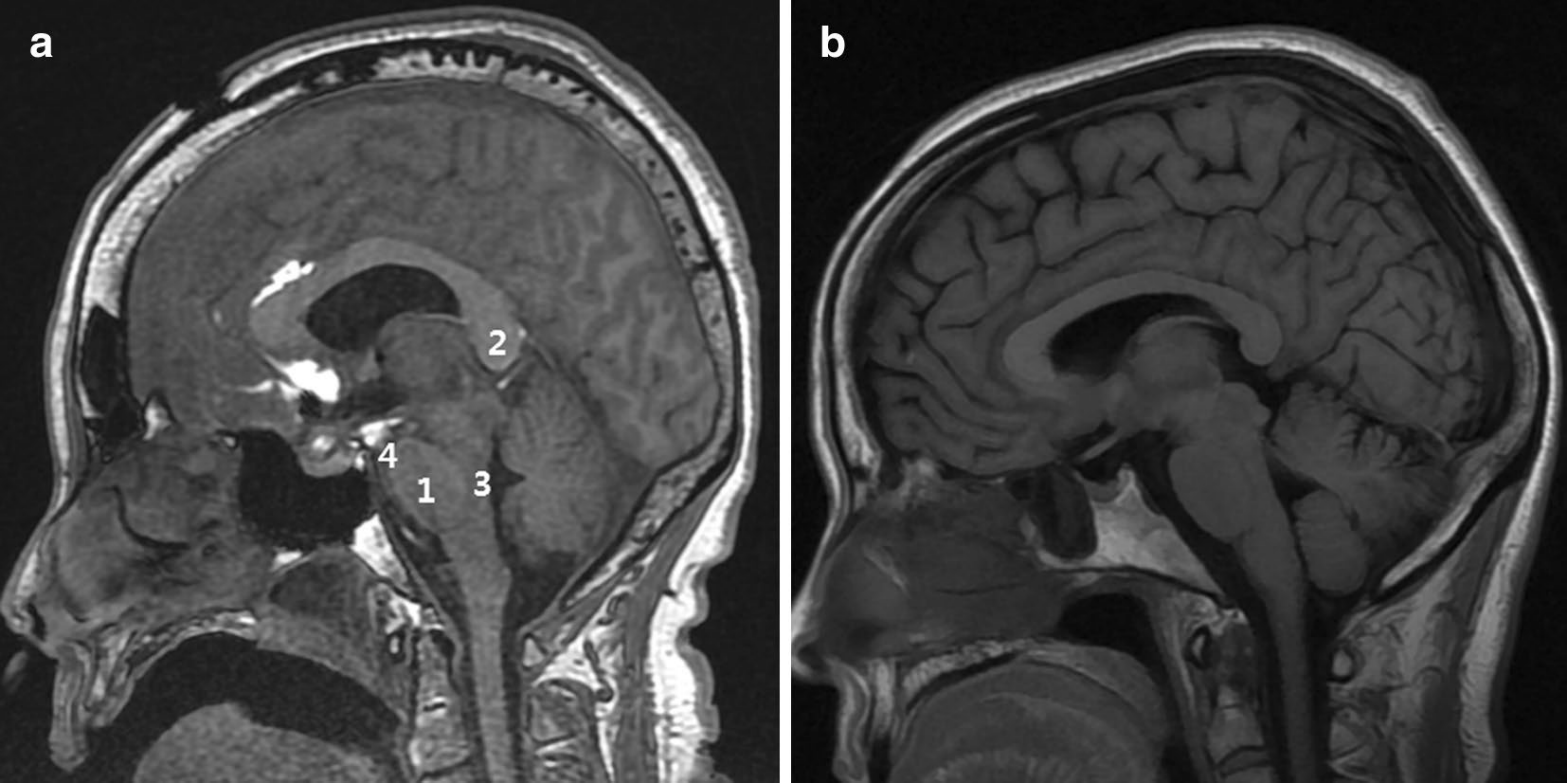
T1-weighted mid-sagittal MRI performed on post-operative Day 5 (a). Compared with age-matched normal brain MRI (b), it demonstrated *1* an oval shaped pons, *2* downward migration of the splenium, *3* vertical shaped anterior wall of the 4th ventricle and *4* narrowing of the interpeduncular and prepontine cisterns
